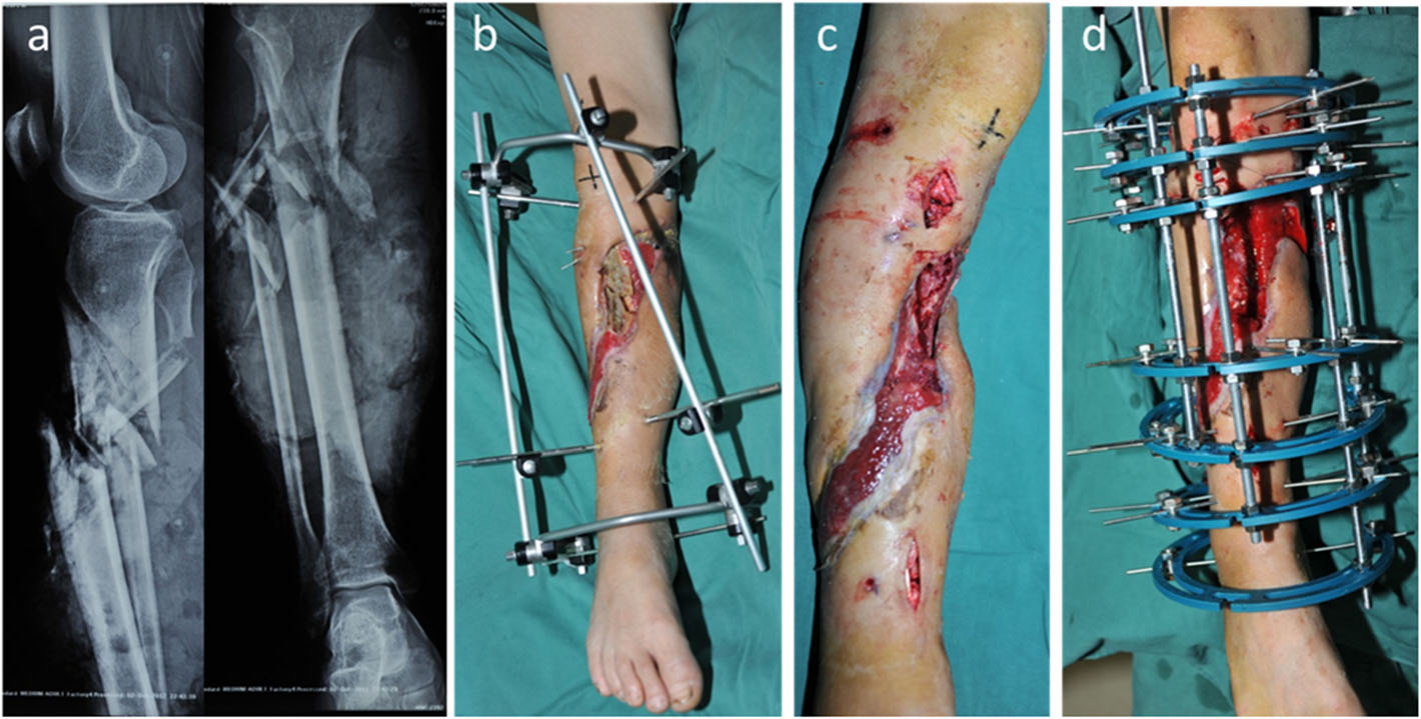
a Radiograph of case no. 27 who was involved in a traffic accident resulting in right tibia open fracture and Gustilo IIIB. b Three weeks later, the patient was transferred to our center and there was lager soft tissue defects associated with facture. c-d The patient underwent the first debridement in our hospital 23 days after injury, and the previous external fixator was replaced with Ilizarov fixation. The wound was open after the necrotic soft tissue and bone was completely debrided, and treated with soft tissue distraction
